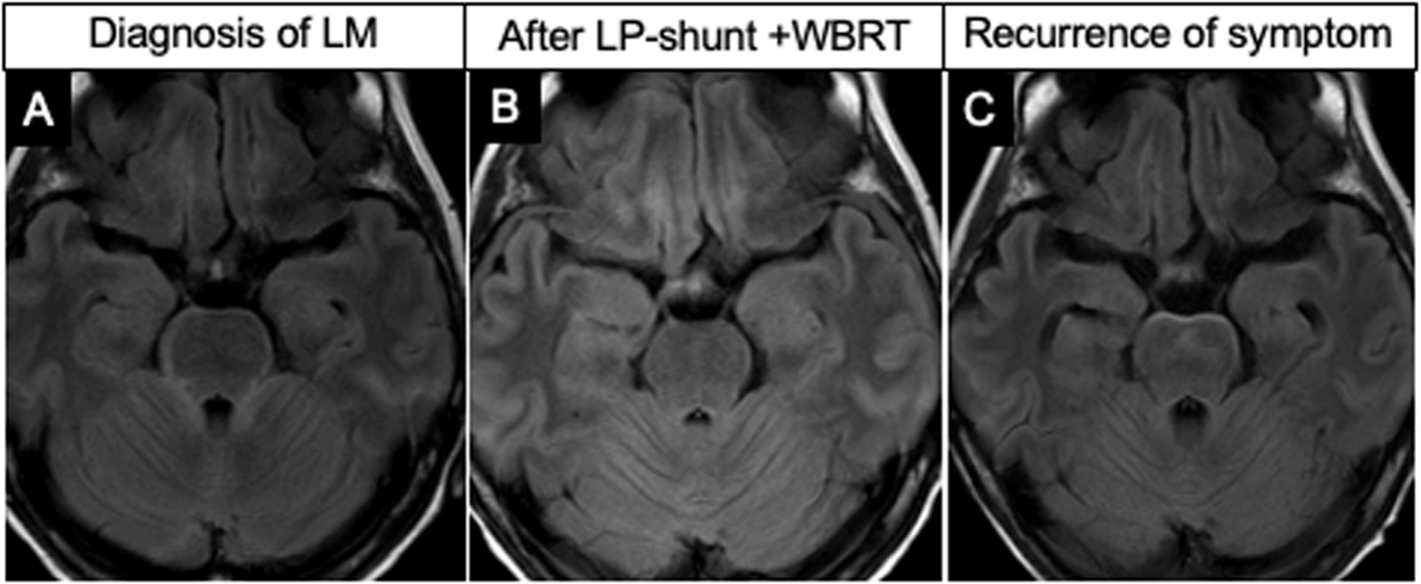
FLAIR images show the bloomy rind sign (Fig. 3a) and demonstrate its disappearance after LP-shunting and WBRT (Fig. 3b). A subsequent brain MR image shows its reappearance with deteriorated neurological symptoms 2 months later (Fig. 3c)
